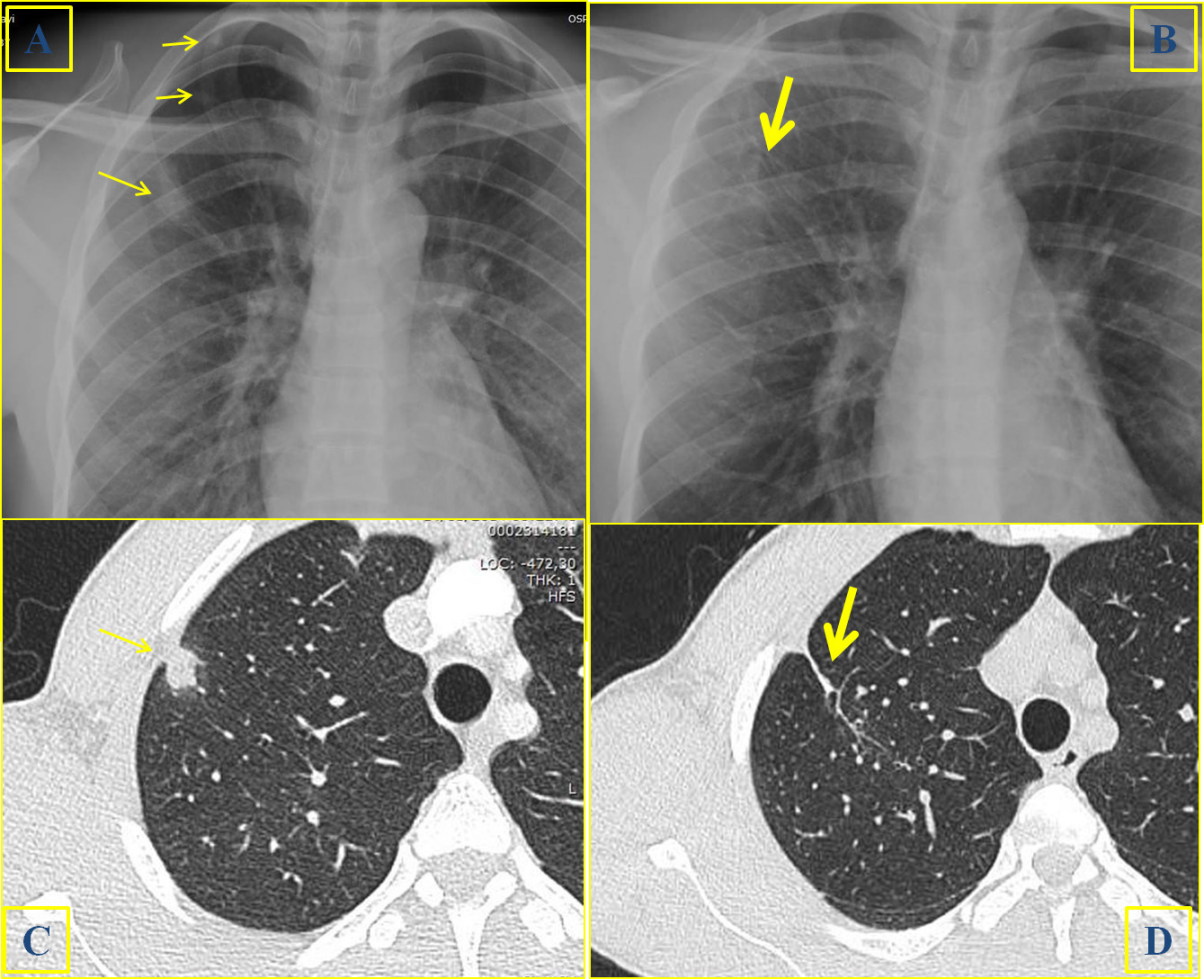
A 27-year-old male with pulmonary schistosomiasis. A prevalently solid nodule (arrow) can be identified at baseline CR (1A) and HRCT (1C); two additional nodules can be recognized in the apical region at CR (1A). The CR (1B) and HRCT (1D) scan acquired 3 weeks later, after medical treatment with praziquantel, showed the absence of any residual disease. Thin parenchymal striae (thick arrow) can be visualized, representing a parenchymal scar.
